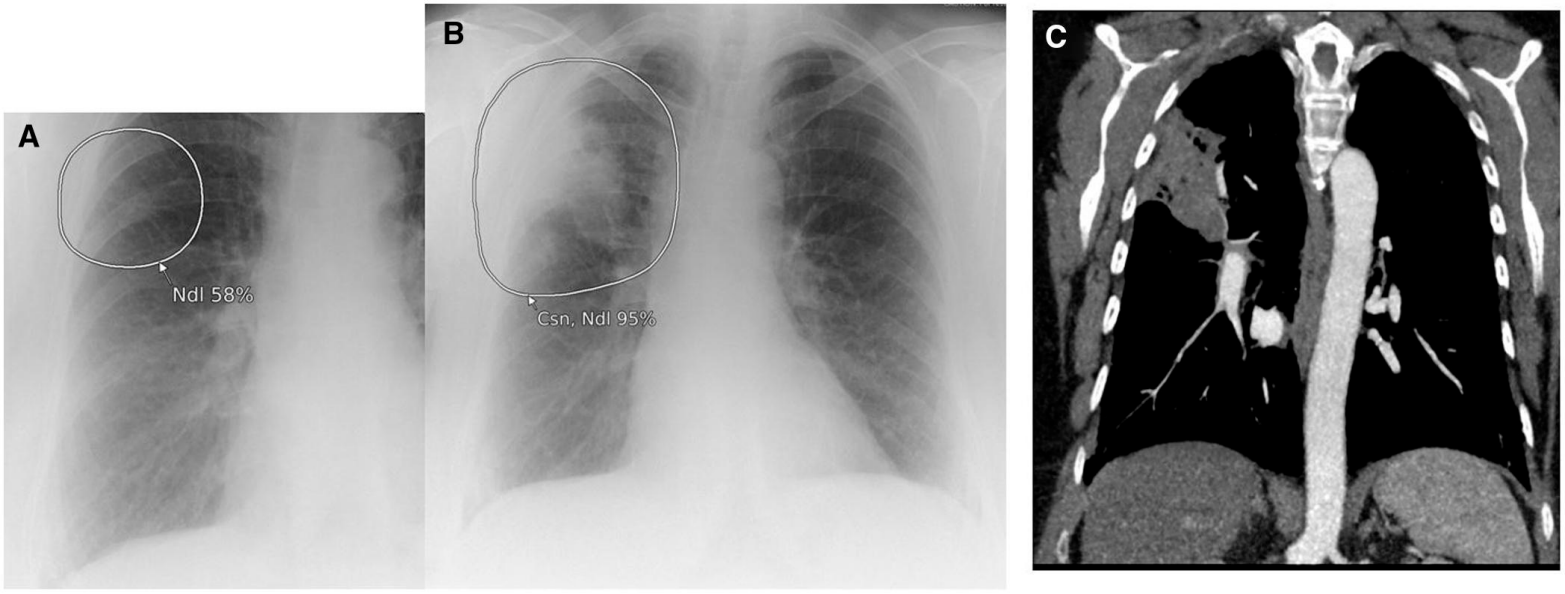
A 62-year-old female with a history of smoking and a wheezy cough underwent a CXR (A) reported as NAD. The AI software highlights suspicion of a nodule in the right upper lobe (white circle). Five years and 5 months later, the patient returns for a CXR (B) due to a persistent hoarse voice and cough, reported as likely consolidation in right upper lobe with a CT suggested to rule out mailgnancy. The AI software had similar suspicion of consolidation and/or nodule secondary on image B. A same day CT scan (C) demonstrates a right upper lobe mass, confirmed as stage IV LC. AI = artificial intelligence; CXR = chest radiograph; NAD = no abnormility detected.
